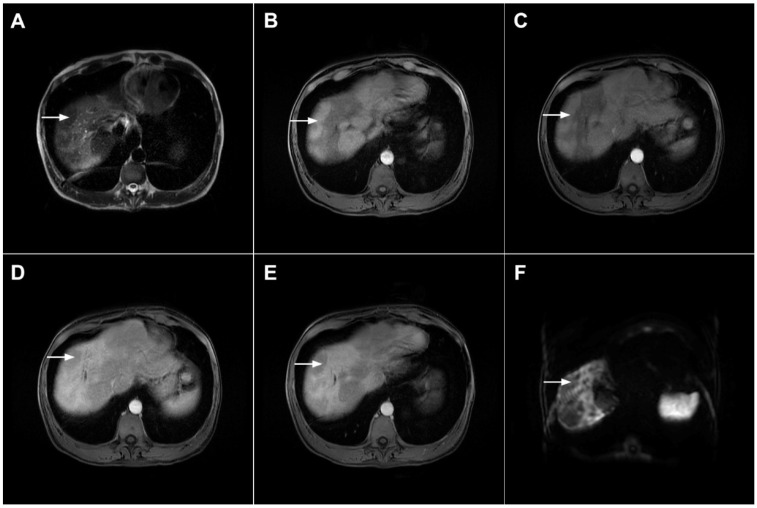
Focal confluent fibrosis in a 42-year-old man with long-standing primary sclerosing cholangitis. The T2-weighted image (A) shows a band like a slightly hyperintense lesion (*arrow*) at the dome of the liver. On the plain T1-weighted image (B) the lesion (*arrow*) is hypointense without arterial vascularity (C) while homogeneous progressive enhancement is seen in the portal venous (D) and delayed phases (E). The lesion (*arrow*) shows high signal intensity in DWI (F).
